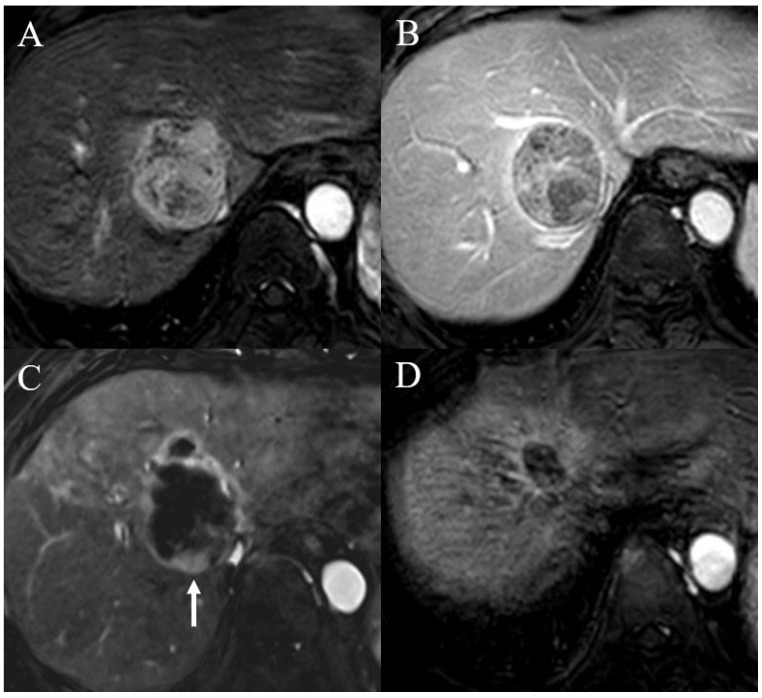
Case of a man who underwent transarterial radioembolization (TARE) for hepatocellular carcinoma. (A,B) Pre-procedure liver dynamic magnetic resonance imaging revealed a 5.6 cm hepatocellular carcinoma in segment VII. (C) On follow-up liver dynamic magnetic resonance imaging at 3 months after TARE, equivocal arterial enhancement (arrow) was noted at the inferior aspect of the treated lesion. The patient was followed up without any additional treatment at the discretion of the referring physician. (D) At 1 year after TARE, the treated lesion was markedly decreased in size, with radiation-related change in the adjacent hepatic parenchyma. The patient was followed up until recently and has remained free of recurrence for 66 months after TARE.
